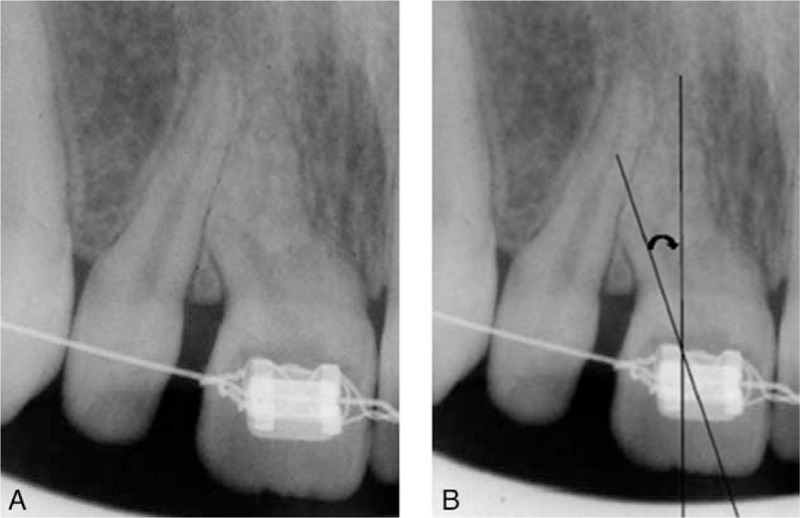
A. Maxillary central incisors; preoperative radiograph showing an S-shaped canal. B. Based on Schneider's method an angle of deviation of 20° was measured.
